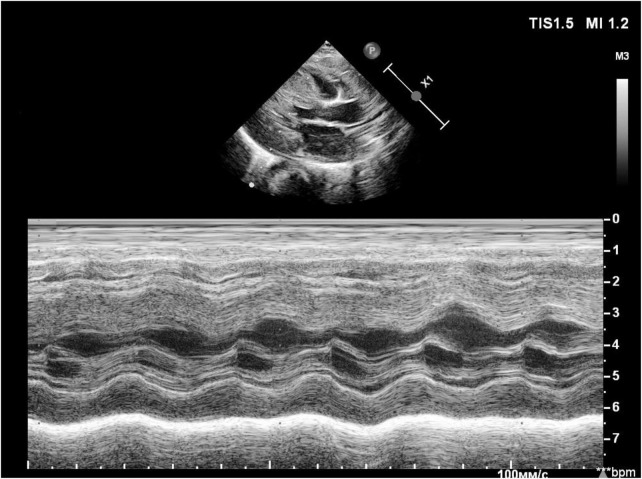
Cardiac ultrasound of the proband.
